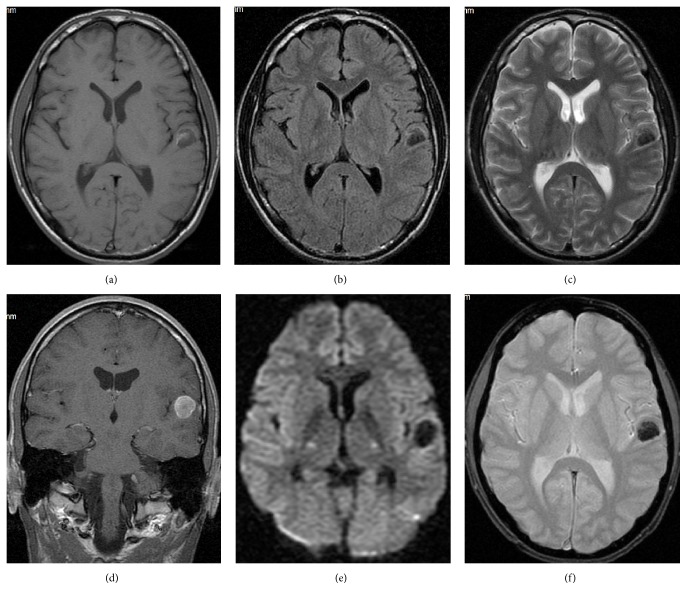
A 16-year-old boy with osteosarcoma of femur. A single calcified metastasis in the left temporal lobe. MRI; SE/T1WI before (a) and after gadolinium-based contrast material injection (d); FLAIR (b); FSE/T2WI (c); DWI (e); GRE/T2*∗*WI (f).
